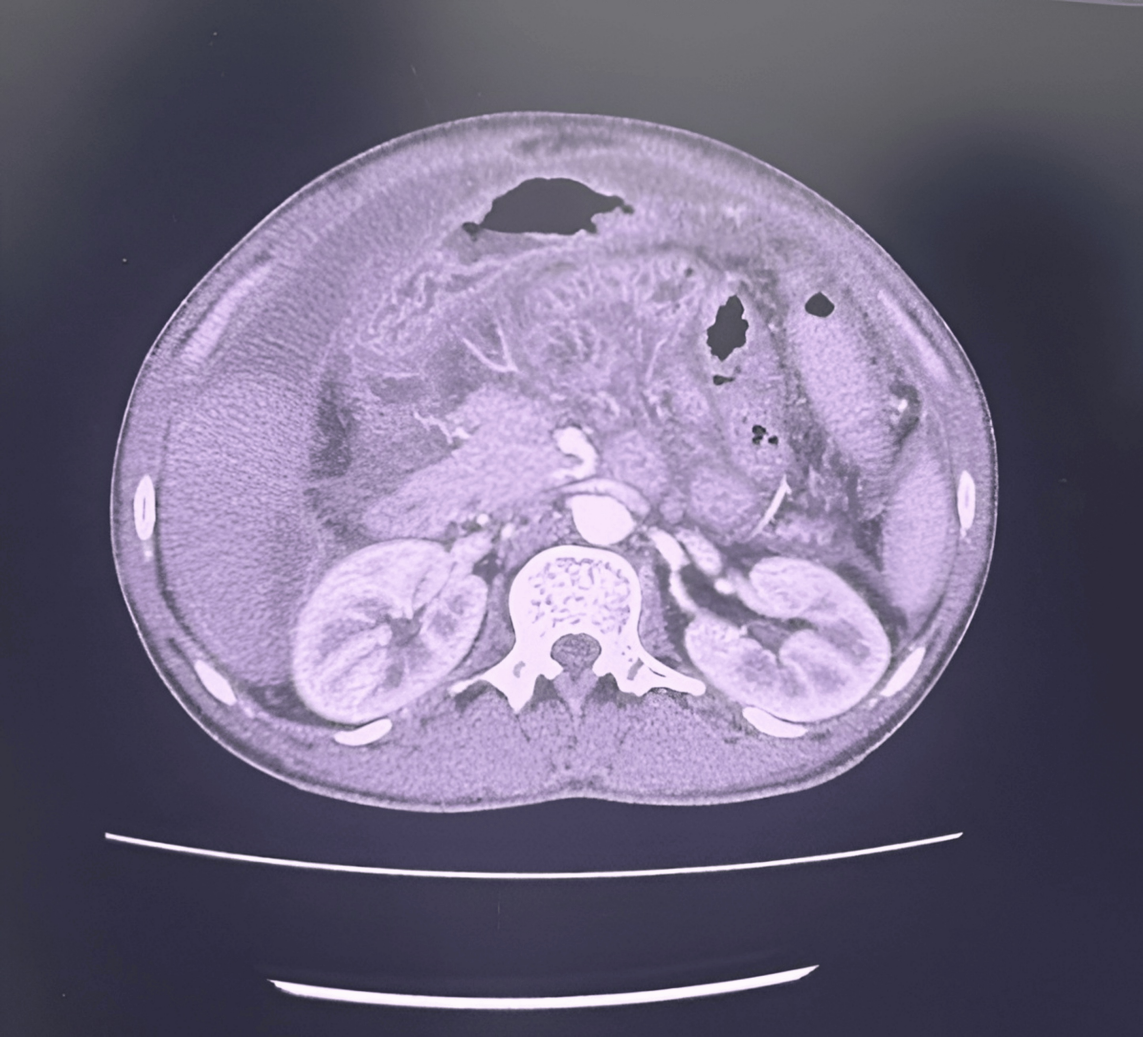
Contrast-enhanced computed tomography of the abdomen demonstrating gross ascites.
